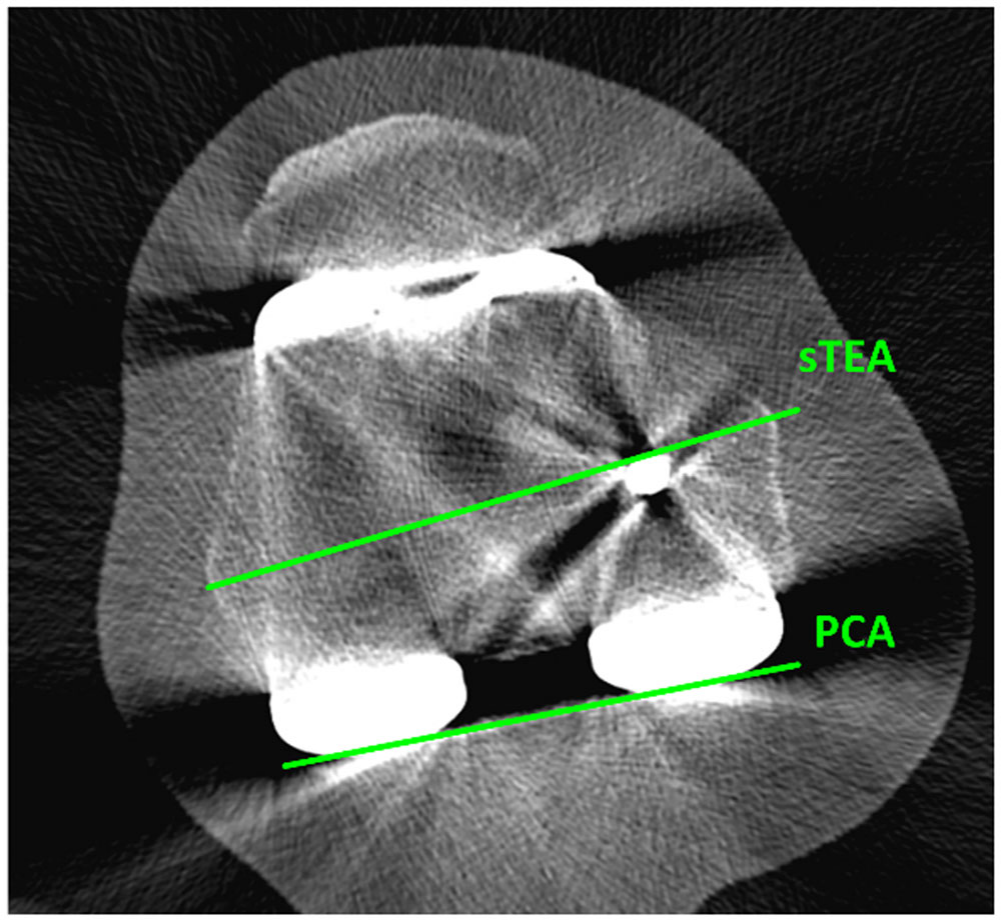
CT femoral component rotation measurement in the axial plane. CT, computed tomography; sTEA, surgical transepicondylar axis.
